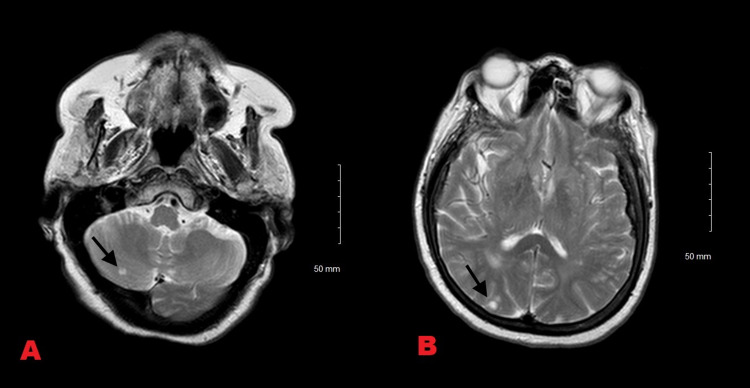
A. MRI brain showing a nodule in the right cerebellar hemisphere (arrow). B. Showing another nodule in the right occipital lobe.
